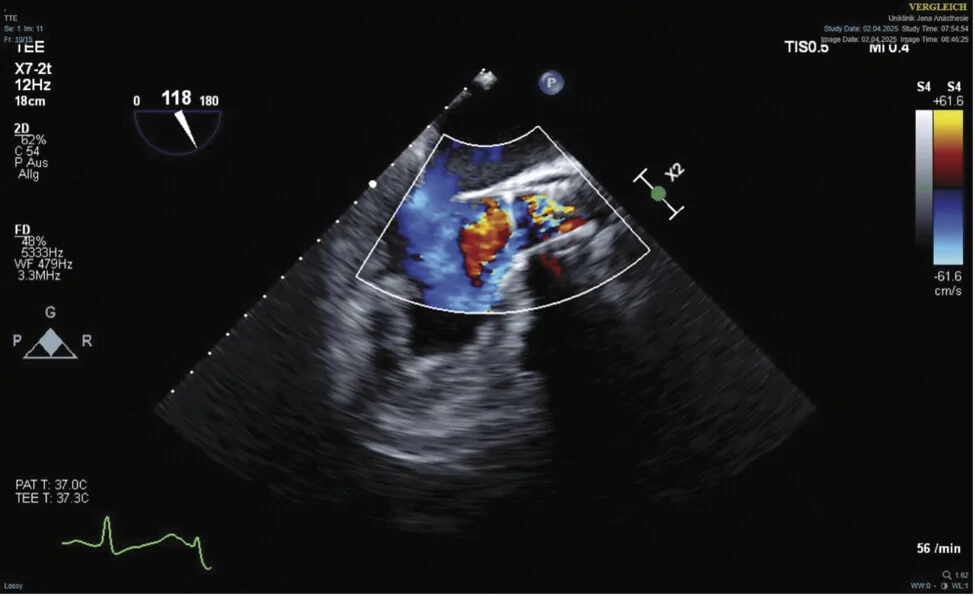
Postoperative echocardiogram showing no signs of paravalvular leakage or any abnormalities.
